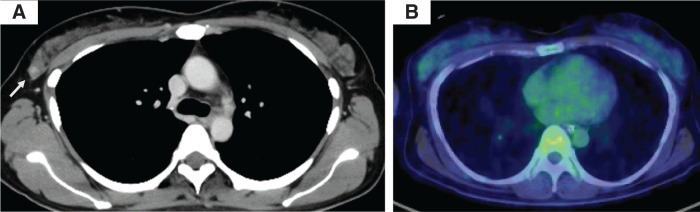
Retrospective evaluation of preoperative breast imaging. (A) Contrast-enhanced CT revealed a subtle, enhancing mass lesion in the outer quadrant of the right breast (arrow). (B) PET-CT showed faint FDG uptake in the corresponding area; however, this was not considered significant at the time of the preoperative assessment.
